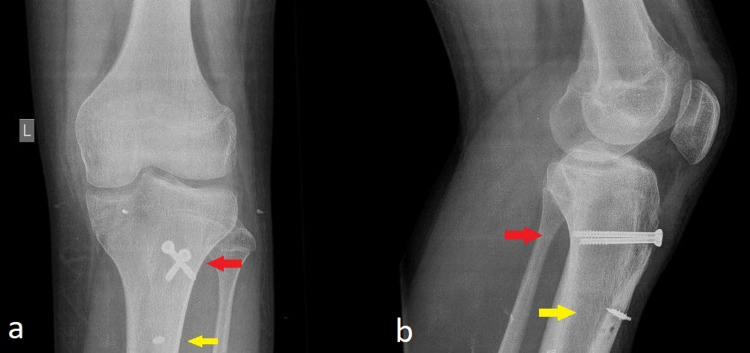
AP (a) and lateral (b) radiographs at five weeks postoperatively The two cannulated screws (red arrows) and the 5 mm anchor below the tibial tubercle (yellow arrows) are noted. AP - anteroposterior
